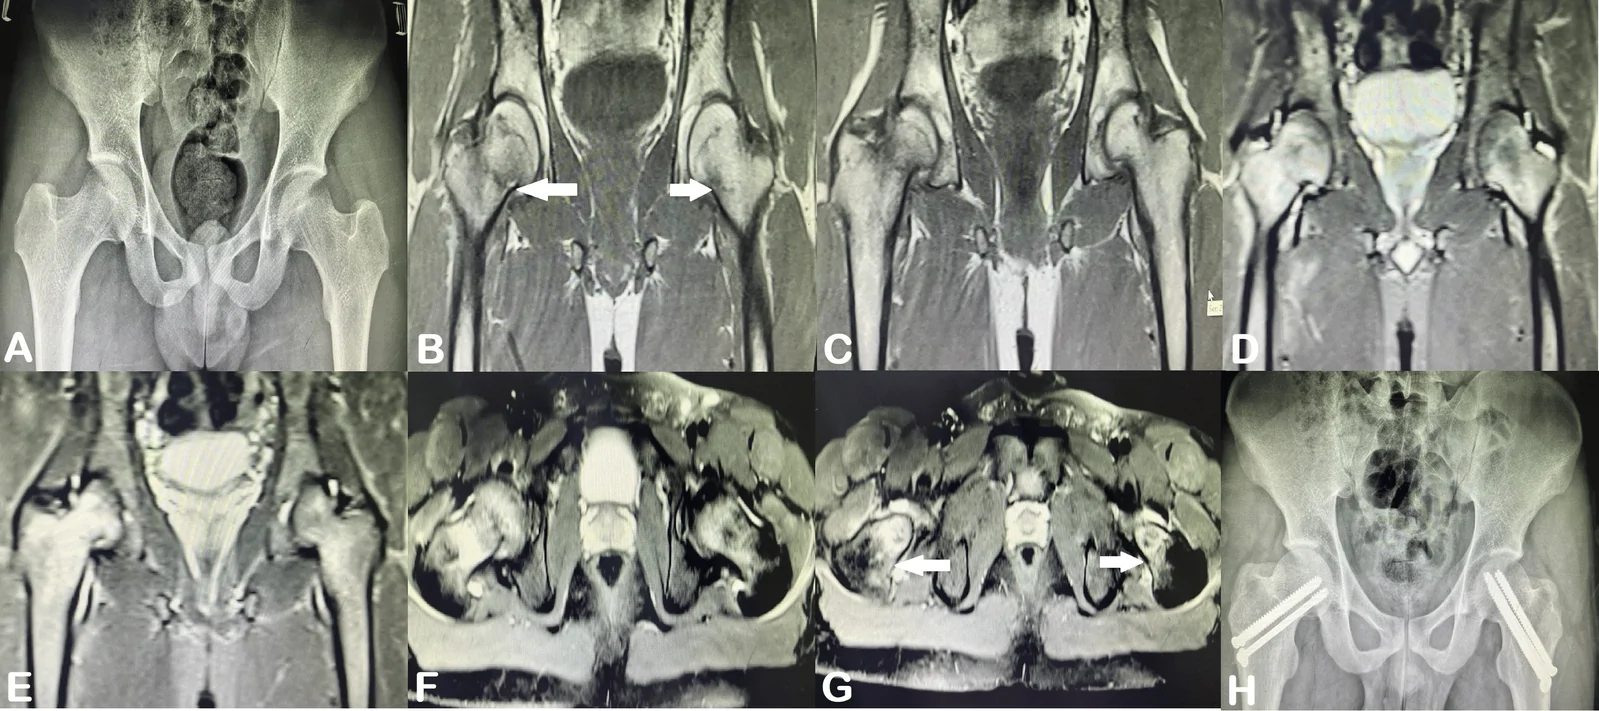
Pelvis radiograph of a 23-year-old male (A) AP pelvis radiograph obtained at the initial presentation of a new 23-year-old male military recruit complaining of bilateral hip pain, showing no obvious pathology. (B, C) T1 coronal bilateral hip MRI images of the same patient showing an obvious fracture line on the right neck of femur with signs of bone edema in the compression aspect of the left neck of femur (white arrow). (D, E) T2 coronal hip MRI images showing obvious bone edema in both necks of femur. (F, G) T2 axial MRI images of both hips showing no obvious fracture line on either side, with significant bilateral neck of femur bone edema representing bilateral neck of femur stress fracture (white arrow). (H) Postoperative two-month follow-up AP pelvis radiograph of the same patient showing the fixation of both fractures by cannulated screws without further displacement. AP, anteroposterior.
